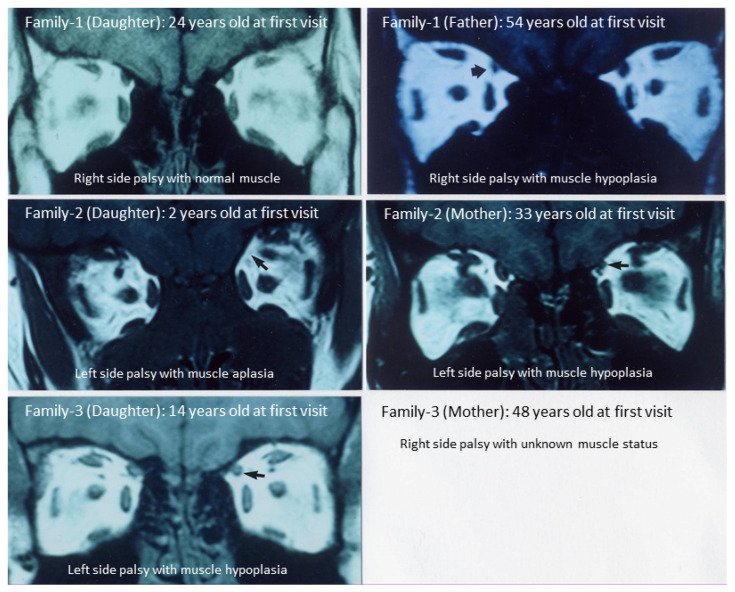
Coronal sections of magnetic resonance imaging in patients with idiopathic superior oblique muscle palsy in three families. One patient (Family 2, Daughter) shows superior oblique muscle aplasia (arrow), while three patients show hypoplasia of the muscle (arrows). Modified from reference [9].
